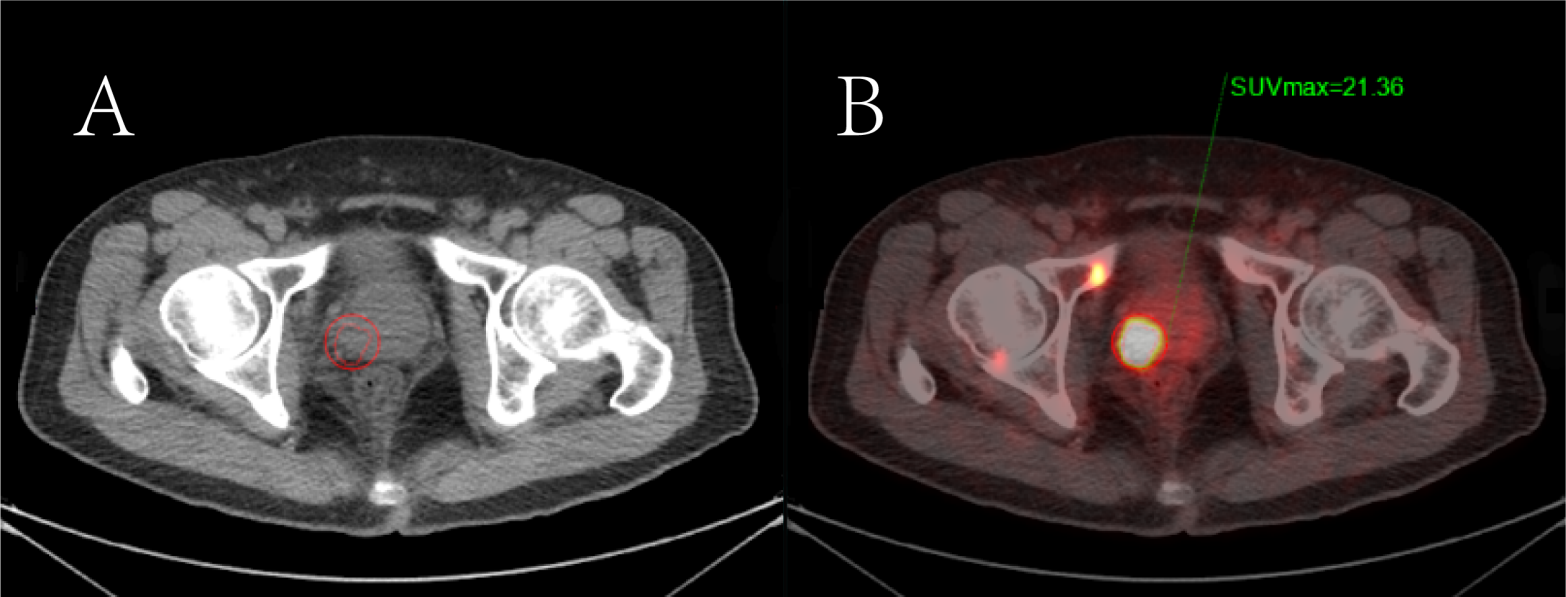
The semi-quantitative parameters of the primary prostate tumor measured by the 3D delineation method in [^18^F]PSMA-1007 PET/CT imaging. (A) Primary prostate cancer is shown on CT imaging; (B) The region of interest of the positive prostate lesion was obtained in the axial fusion image (the smaller red circle surrounding the lesion). The maximum standardized uptake value (SUVmax) of the lesion was obtained as 21.36 by the threshold method.
